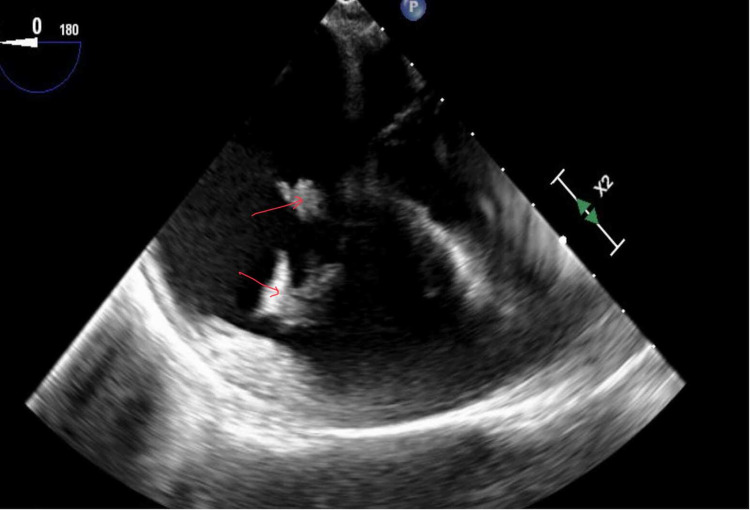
TEE showing lesions on the anterior and septal leaflet of the tricuspid valve TEE: transesophageal echocardiography
